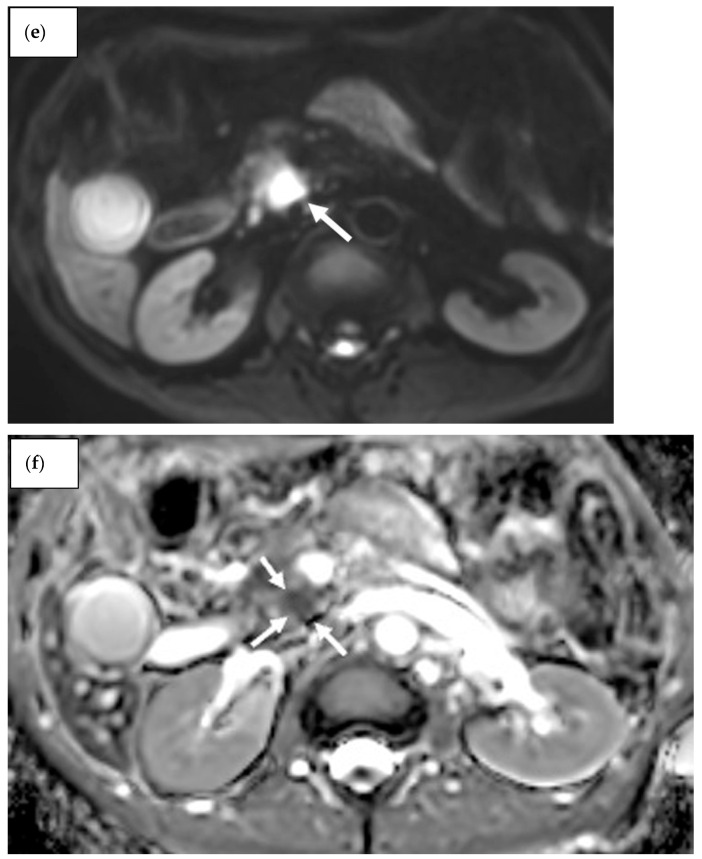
A 67-year-old man with pancreatic ductal adenocarcinoma (PDAC). (a–d) CT images pre-contrast (a) and during the pancreatic phase (b), portal venous phase (c), and equilibrium phase (d). Regions of interest (in black) are manually drawn on the PDAC region and abdominal aorta on pre-contrast (a) and equilibrium-phase (d) images. The calculated contrast enhancement was 38.5 HU and the extracellular volume fraction was 36.1%. (e) Diffusion-weighted imaging (b = 800 s/mm^2^) shows the PDAC region with high signal intensity (arrow). (f) The apparent diffusion coefficient is 0.79 × 10 ^−3^ mm^2^/s (arrows).
